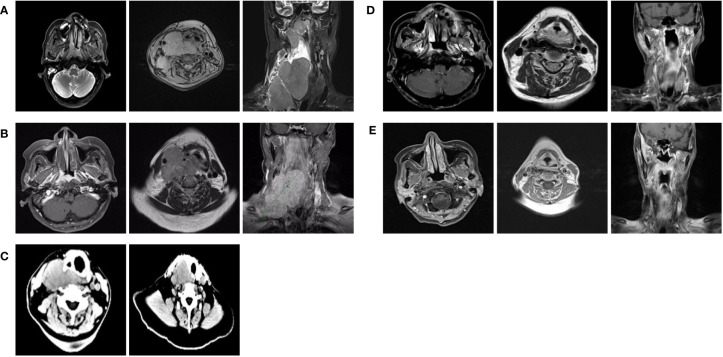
(A) MRI of the nasopharynx and lymph node before treatment (July, 2022). (B) MRI of the nasopharynx and lymph node after 2 two cycles of GPchemotherapy regimen (August, 2022). (C) CT of the lymph node after loading dose of toripalimab along with cetuximab (August, 2022). (D) MRI of the nasopharynx and lymph node after radiotherapy (October, 2022). (E) MRI of the nasopharynx and lymph node performed 2 months after the completion of radiotherapy (December, 2022).
